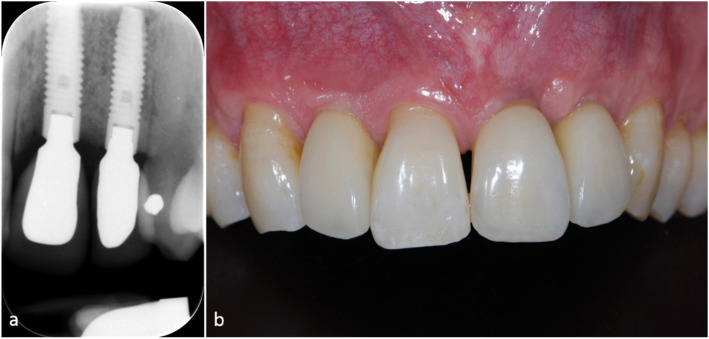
Reference: The apical dental x-ray 10 months after implantation (a) and the consecutive prosthetic reconstruction (b), Courtesy of N. Nänni & D. Thoma, Clinic of Reconstructive Dentistry, University of Zurich
